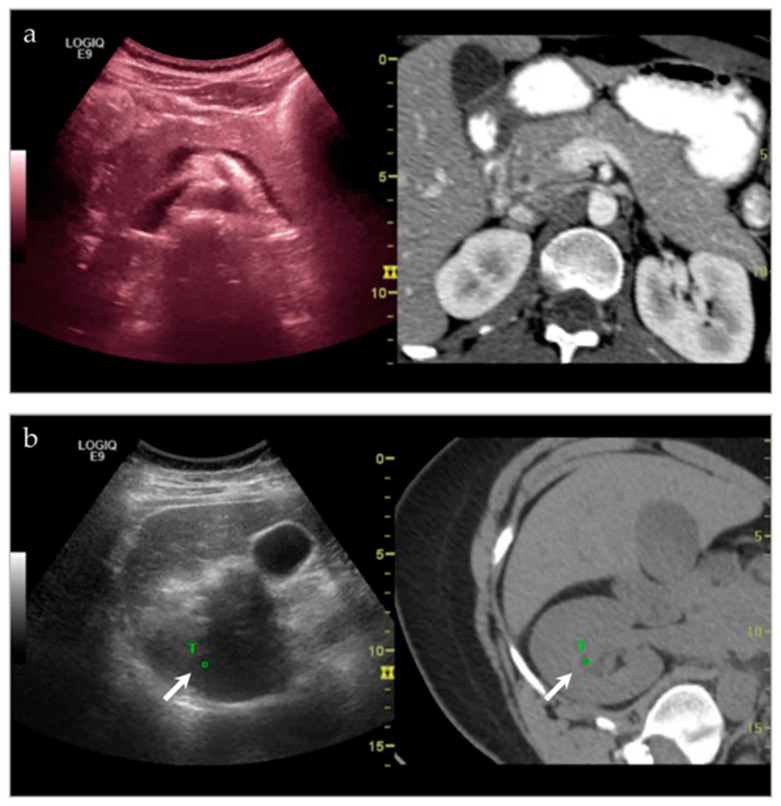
(a) Side-by-side imaging of the ultrasound image fused with the corresponding CT dataset. As the ultrasound moves, the multiplanar reformatted CT (MPR) tracks with it as well, in real time. (b) Target points are chosen on both the ultrasound and MPR CT image, in order to coordinate movement. In this instance, one of the target points (white arrow, T) that is chosen is the right renal pelvis.
